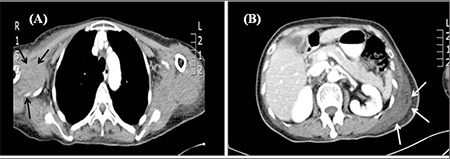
(A) CT scan image demonstrating a subcutaneous haematoma in the right axilla region impinging on the surrouding vessels (black arrows). (B) CT scan image demostrating an intramuscular haematoma in the left lattissimus dorsi muscle (white arrows)
